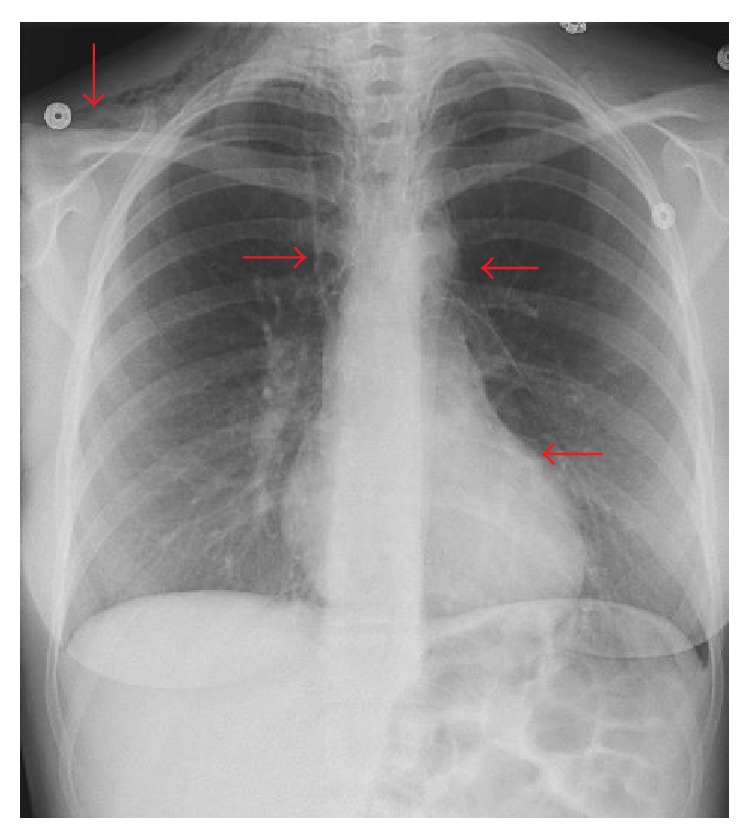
The chest X-ray demonstrates extensive subcutaneous emphysema and pneumopericardium (red arrows).
